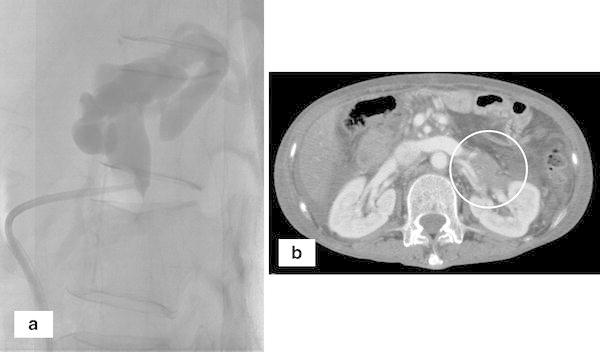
Balloon-occluded retrograde transvenous obliteration. a Before the procedure, there was significant dilation of the splenorenal shunt. b The injection of 20 ml of 5 % ethanolamine oleate iopamidol eliminated flow through the shunt, and a follow-up CT scan on day 3 showed good occlusion of the shunt (*circle*)
